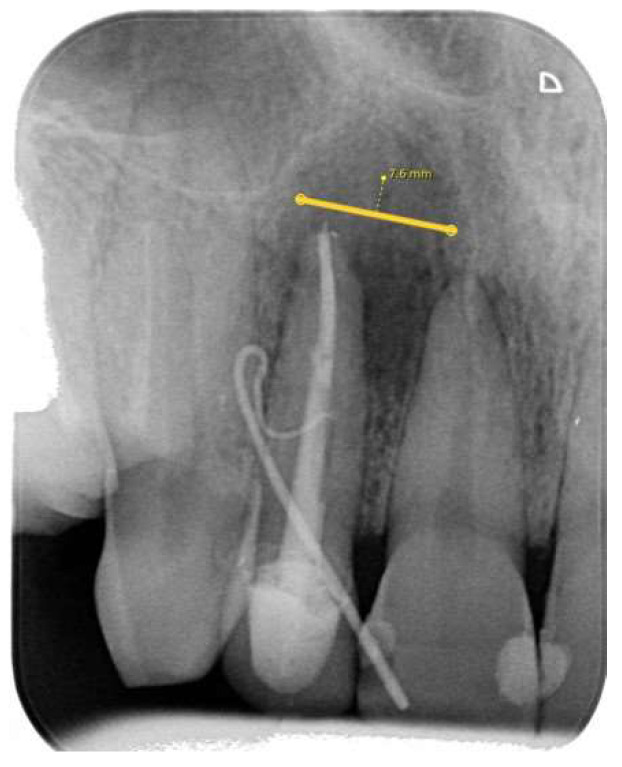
PA radiograph showing the measurement of a periapical lesion related to the maxillary right second incisor.
